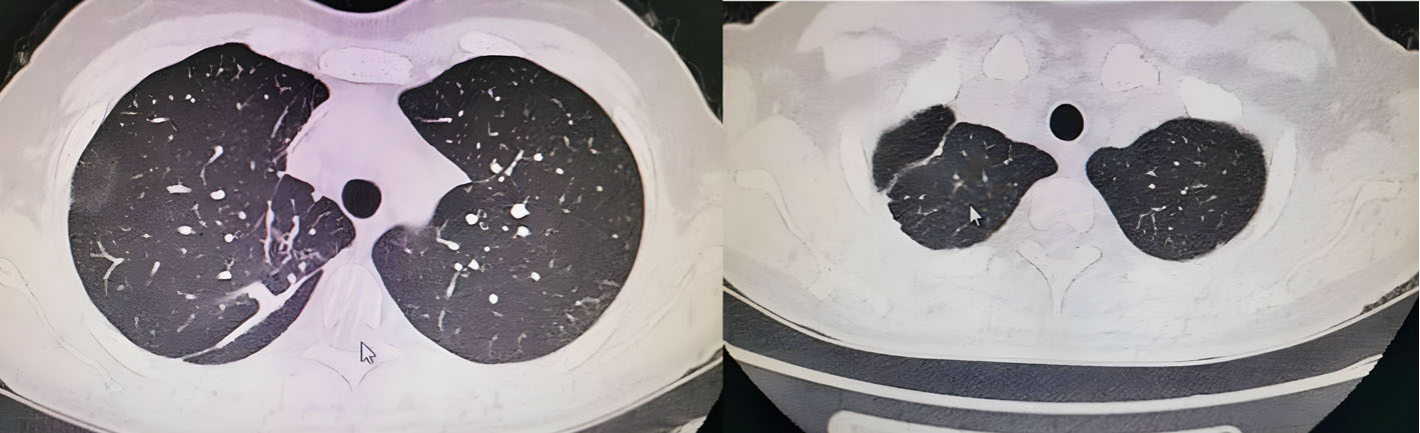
(a) (b) The postoperative lung CT scan for RS3b + S2a after 3 months.
